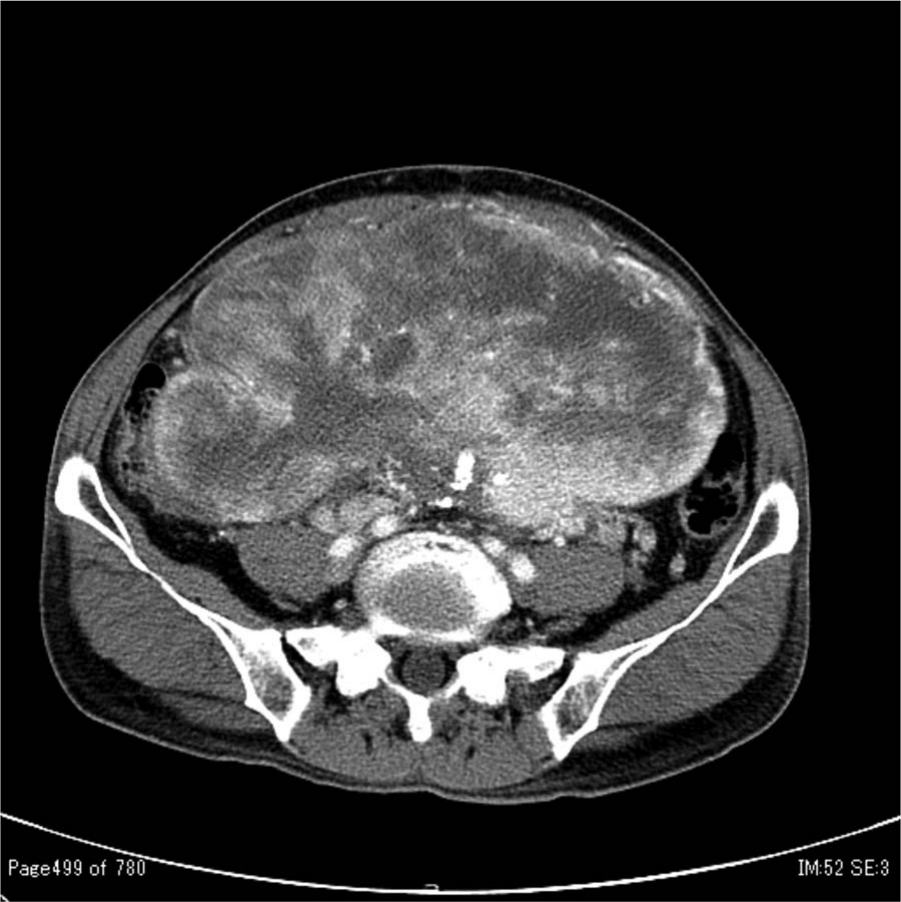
Contrast-enhanced CT showing a large tumour measuring 20 cm in diameter occupying both the abdominal and pelvic cavities.
